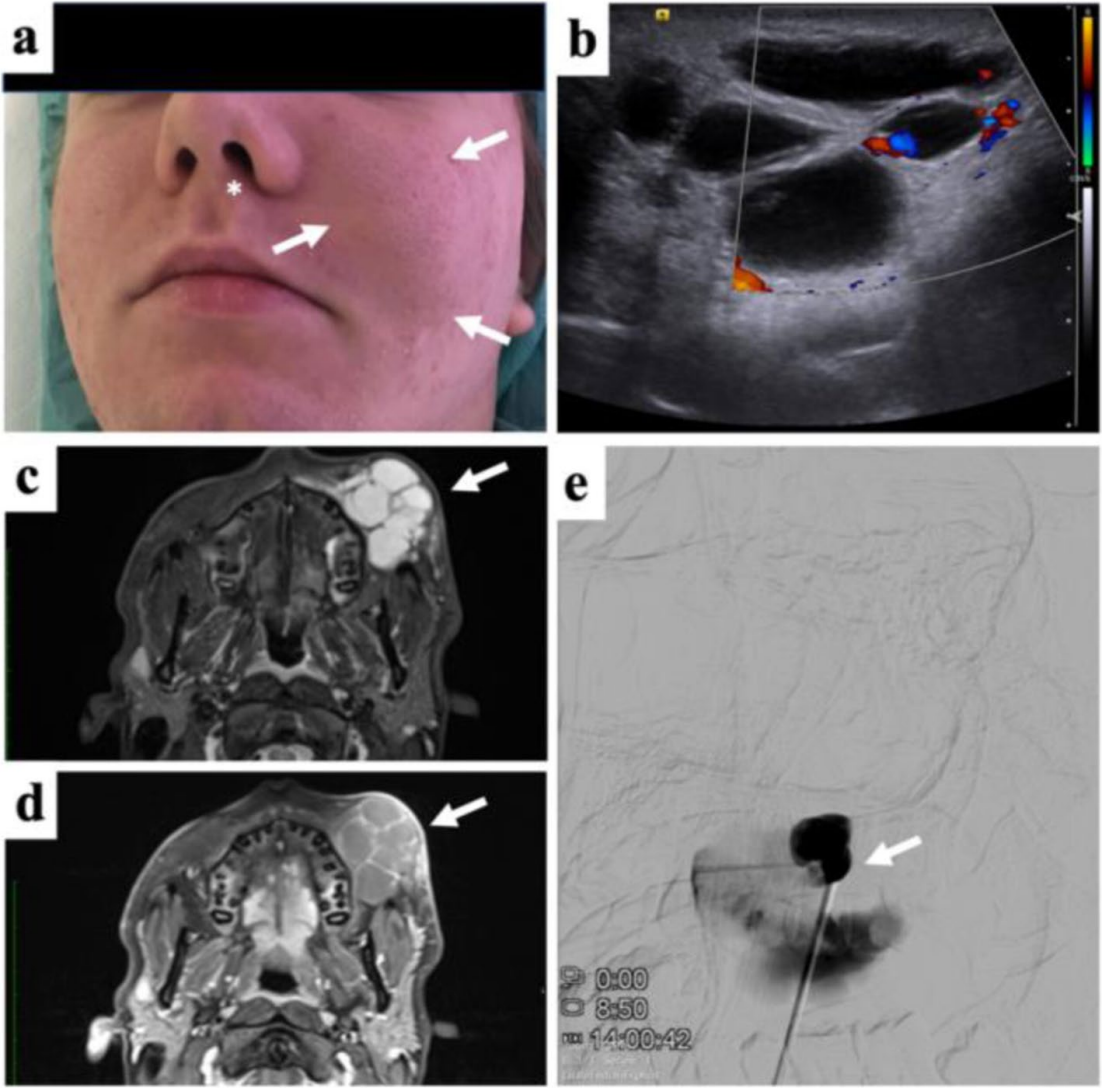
Subcutaneous Lymphatic malformation of the face in an adolescent. a Clinical photograph showing local swelling and moderate discoloration of the cheek (white arrows) and local deviation of the left ala of the nose (asterisk). The patient was suffered from esthetic disfigurement with the mass itself being painless. b Color-coded Duplex ultrasound documents the absence of intralesional flow, only focal vascularization along the septa. c Fat-saturated STIR MRI shows fluid-filled cavities in the subcutaneous tissue of the mixed-type lymphatic malformation (arrow). d Following contrast administration only septal structures show moderate enhancement (arrow). e Percutaneous contrast administration before sclerotherapy reveals partial communication between adjacent lymphatic cysts. Sclerotherapy resulted in subtotal regression of the mass
